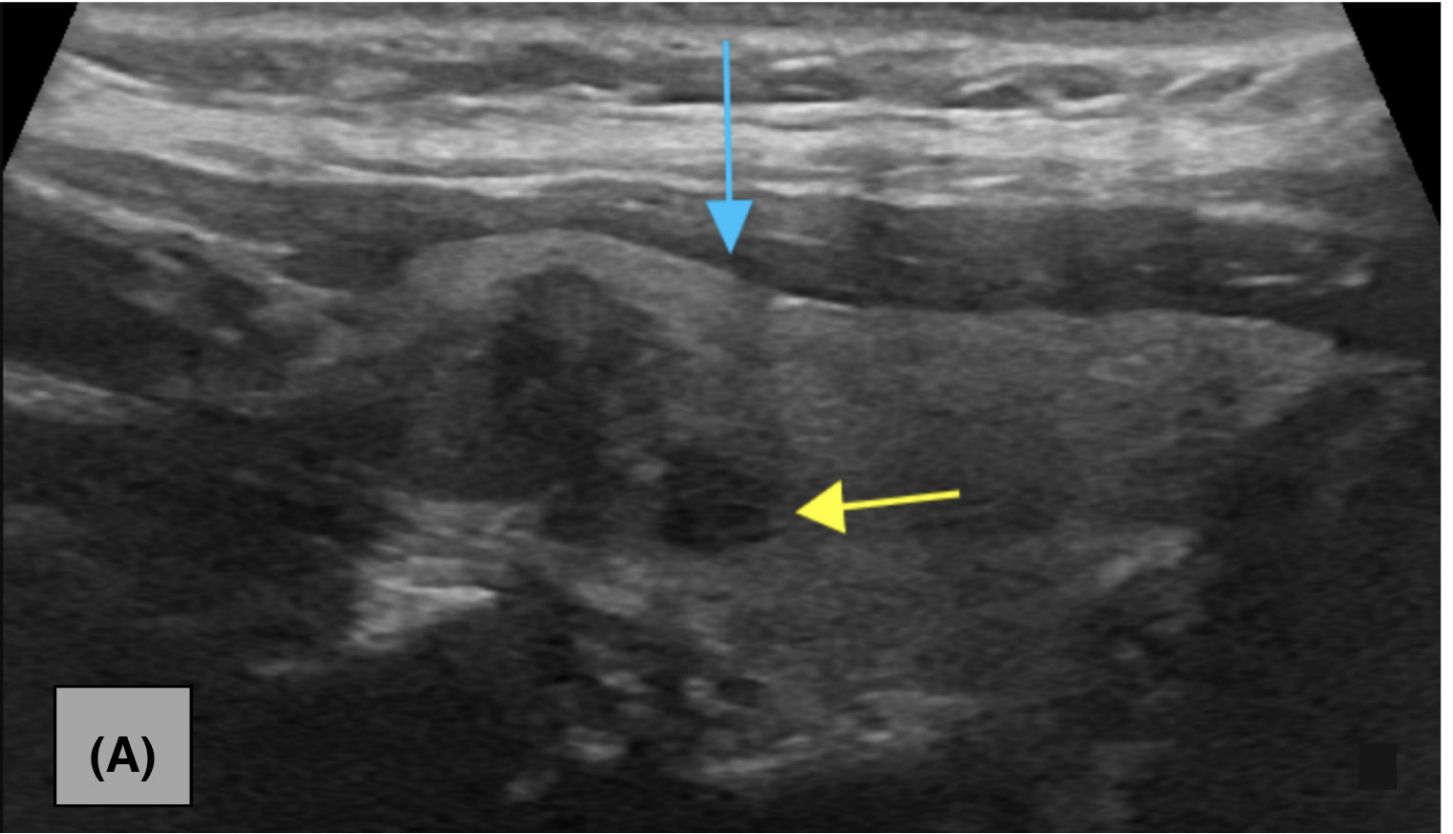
(A) Longitudinal‐axis ultrasound image of the right thyroid lobe demonstrates further nodule enlargement to 1.9 × 1.3 × 0.9 cm. There is a very hypoechoic echotexture of the nodule inferior aspect (yellow arrow) when compared to overlying musculature (blue arrow).
